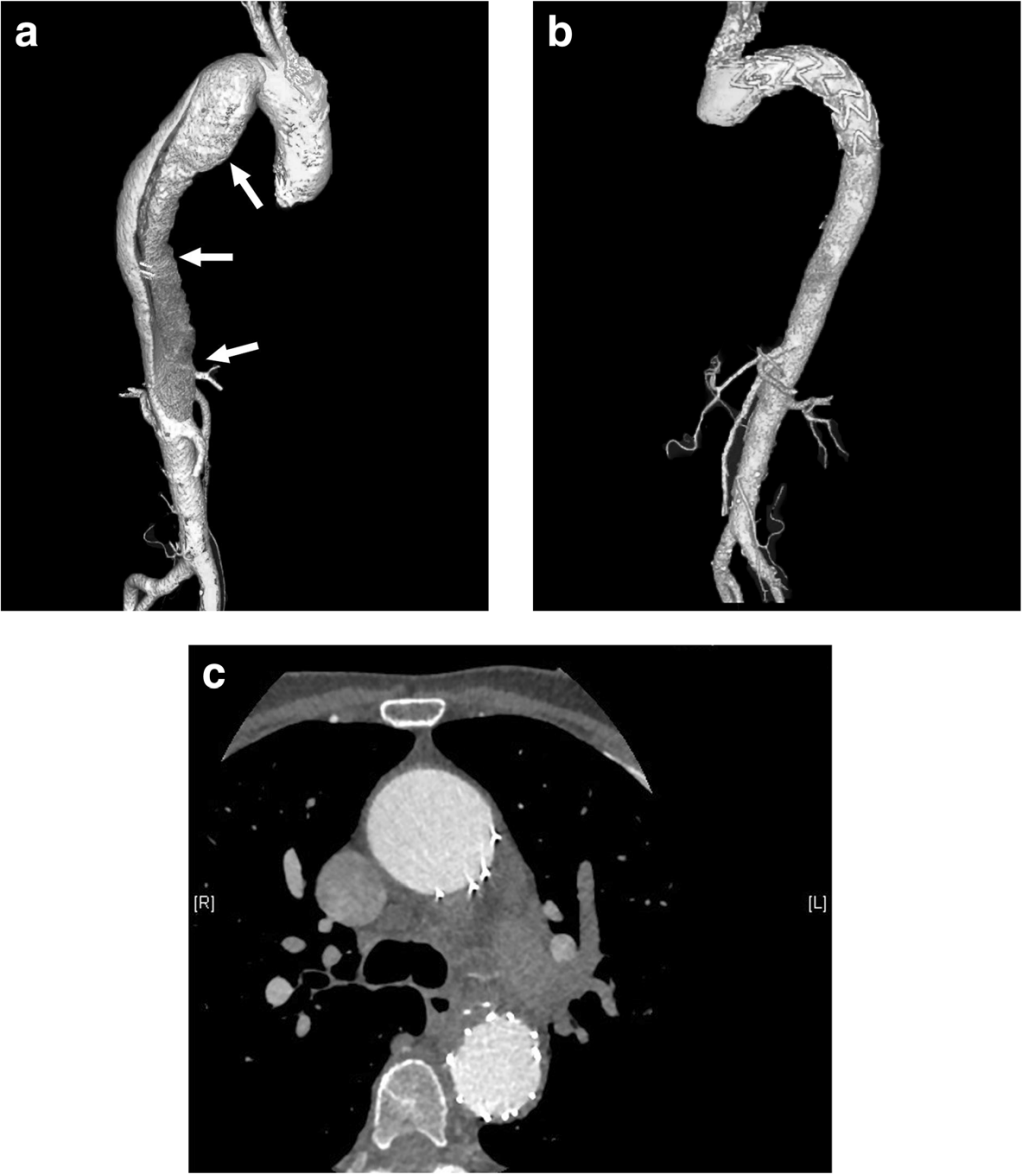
CT angiograms. a Dissection of the descending aorta in 2009 (arrows). b, c Repeat study in 2017 revealed no new dissection after TEVAR. CT: computed tomography; TEVAR: thoracic endovascular aortic repair
